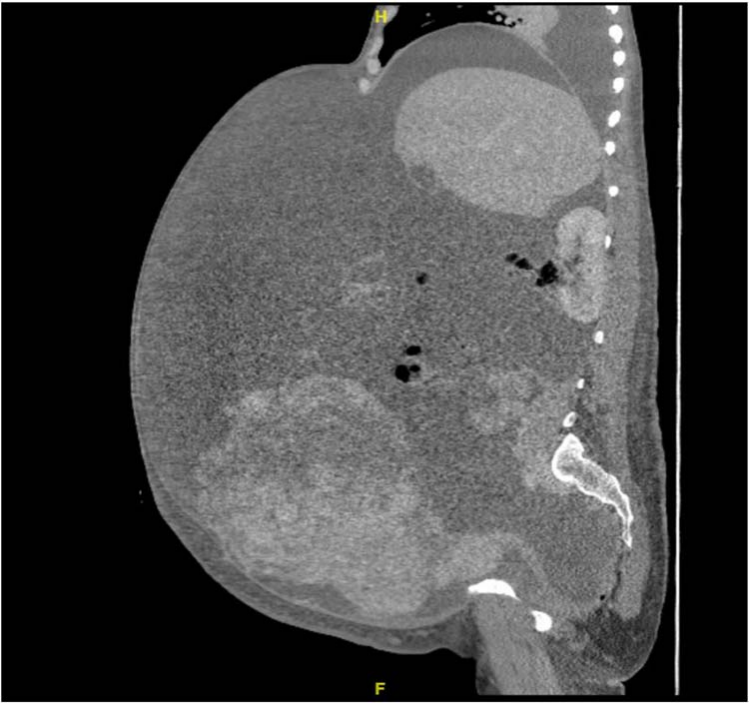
Abdominal CT scan shows huge ascites.
